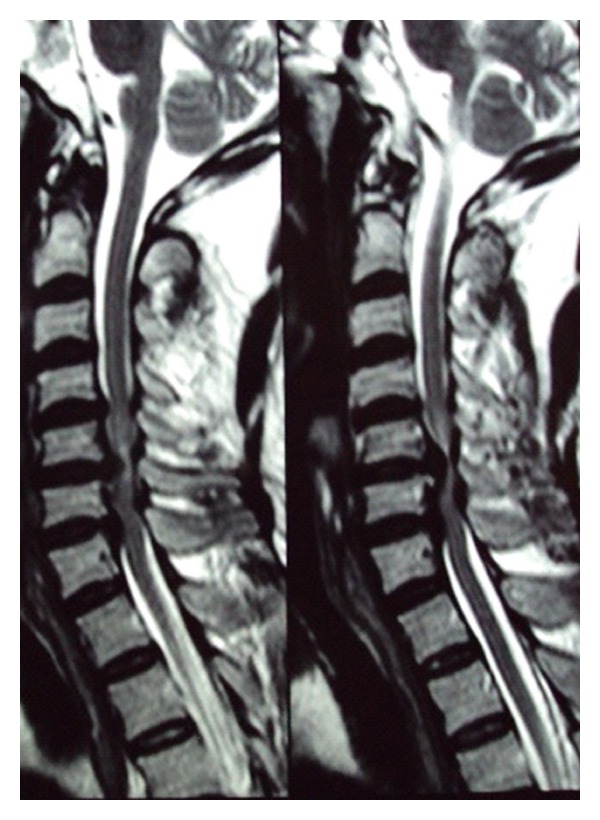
Sagittal MRI image showing C4-C5,C5-C6,C6-C7 disc herniation with caudal migration behind the vertebral body.
